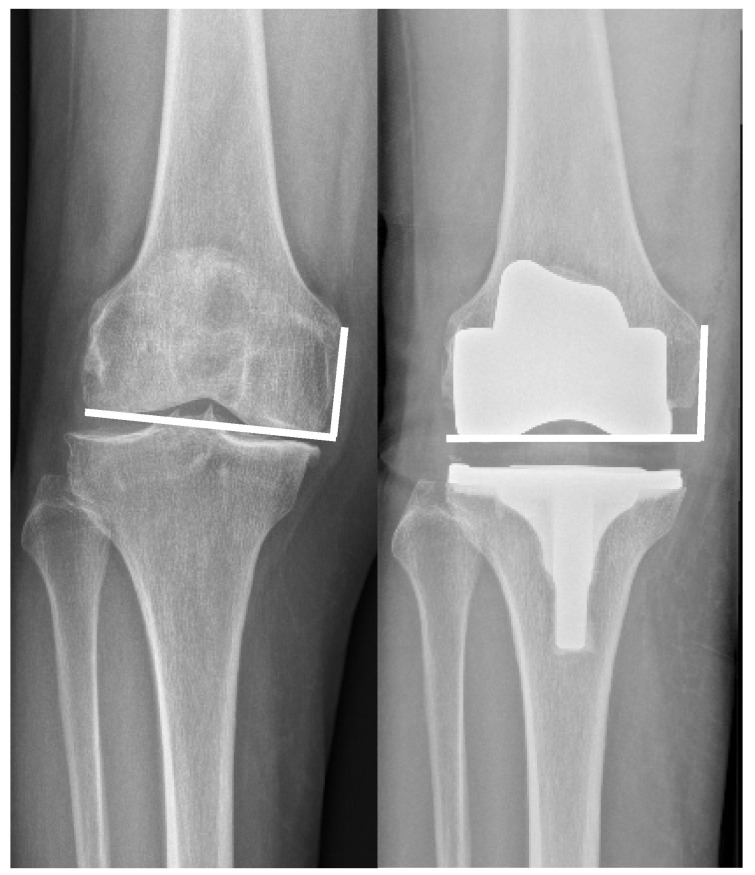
The joint line position was measured on pre- and postoperative anterior–posterior radiographs using the adductor tubercle to joint line distance as previously reported by Hofmann et al. [1].
